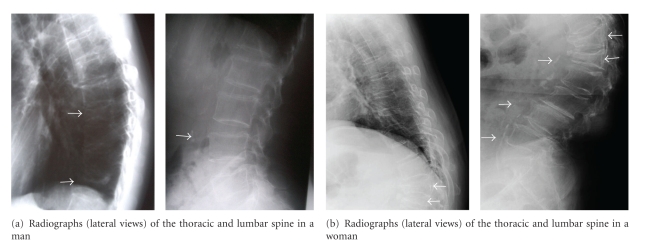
Radiographs (lateral views) of the thoracic and lumbar spine in a man (a) and a woman (b). Abdominal aortic calcification is prominently displayed on routine lateral lumbar spine radiographs as the dense calcium mineral deposits in the aorta of subjects with osteoporotic vertebral fractures.
